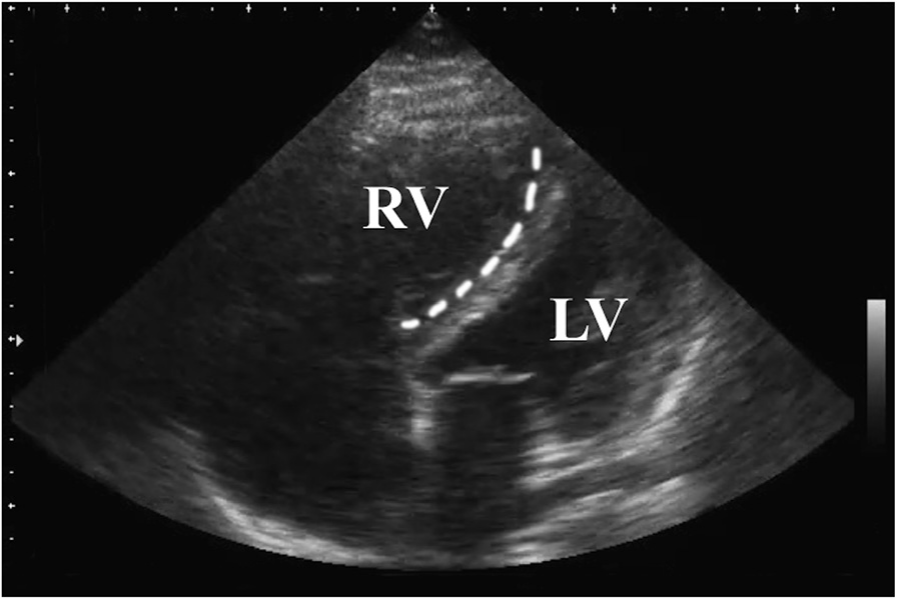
Representative image of pulmonary hypertension by bedside echocardiogram. The right ventricle (RV) is dilated and the intraventricular septum (dashed line) is bowing into the left ventricle (LV). (Courtesy of Geoffrey Hayden, MD).
